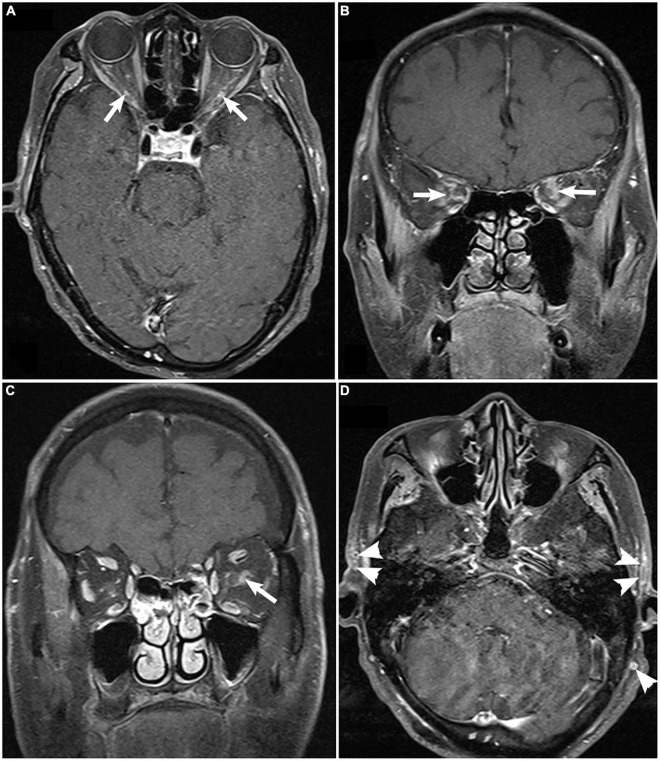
Fat-suppressed contrast-enhanced T1-weighted magnetic resonance imaging of the orbit demonstrating enhancement of bilateral optic nerve sheaths [arrow; (A), axial; (B), coronal], unilateral enhancement of optic nerve sheaths [(C), arrow], and enhancement and thickening superficial temporal artery [(D), arrowhead].
